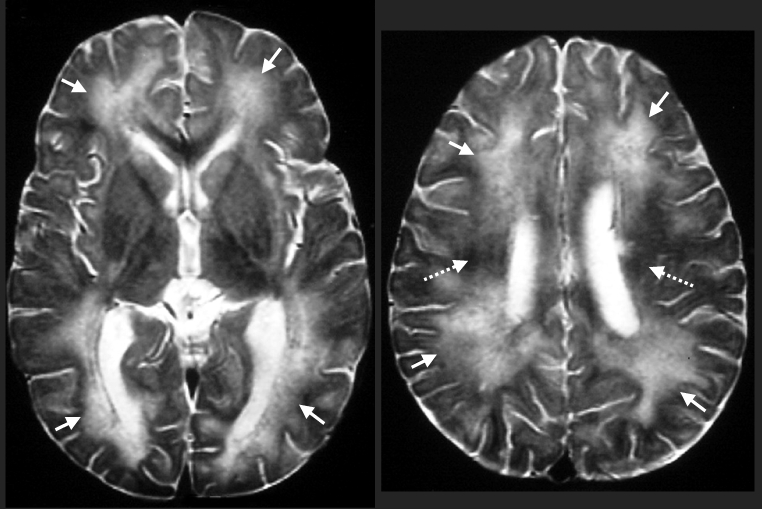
Biopsy-proven *Baylisascaris procyonis* encephalitis in a 13-month-old boy. Axial T2-weighted magnetic resonance images obtained 12 days after symptom onset show abnormal high signal throughout most of the central white matter (arrows) compared with the dark signal expected at this age (broken arrows).
